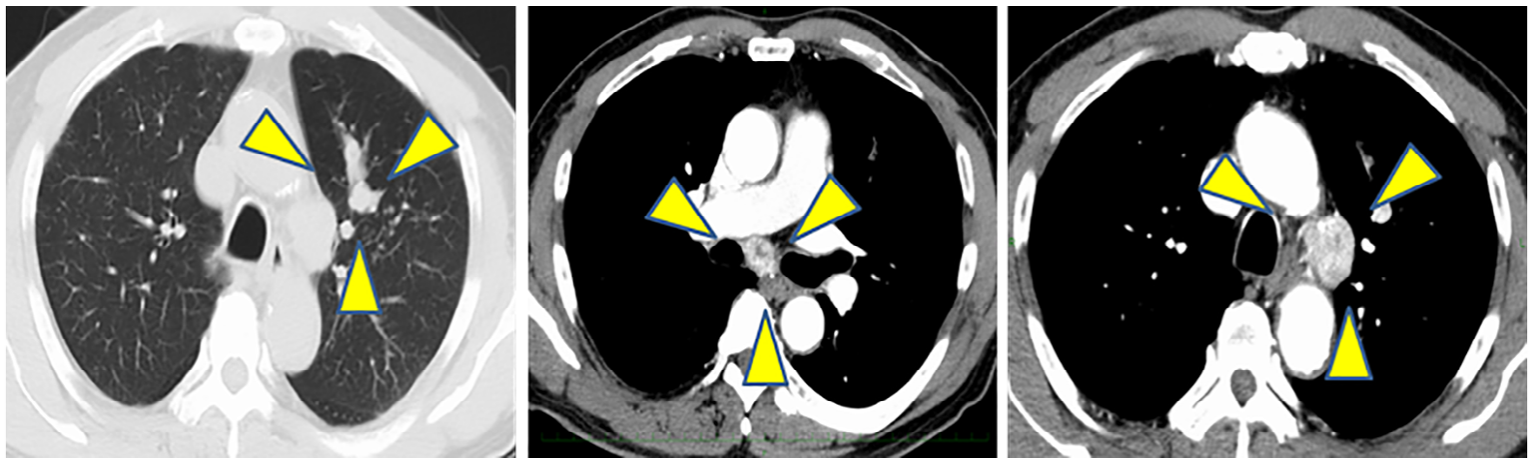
Follow‐up CT findings at 1 year after surgery for mRCC. CT shows a nodule in the upper lobe of the left lung and swelling of the mediastinal lymph nodes.
